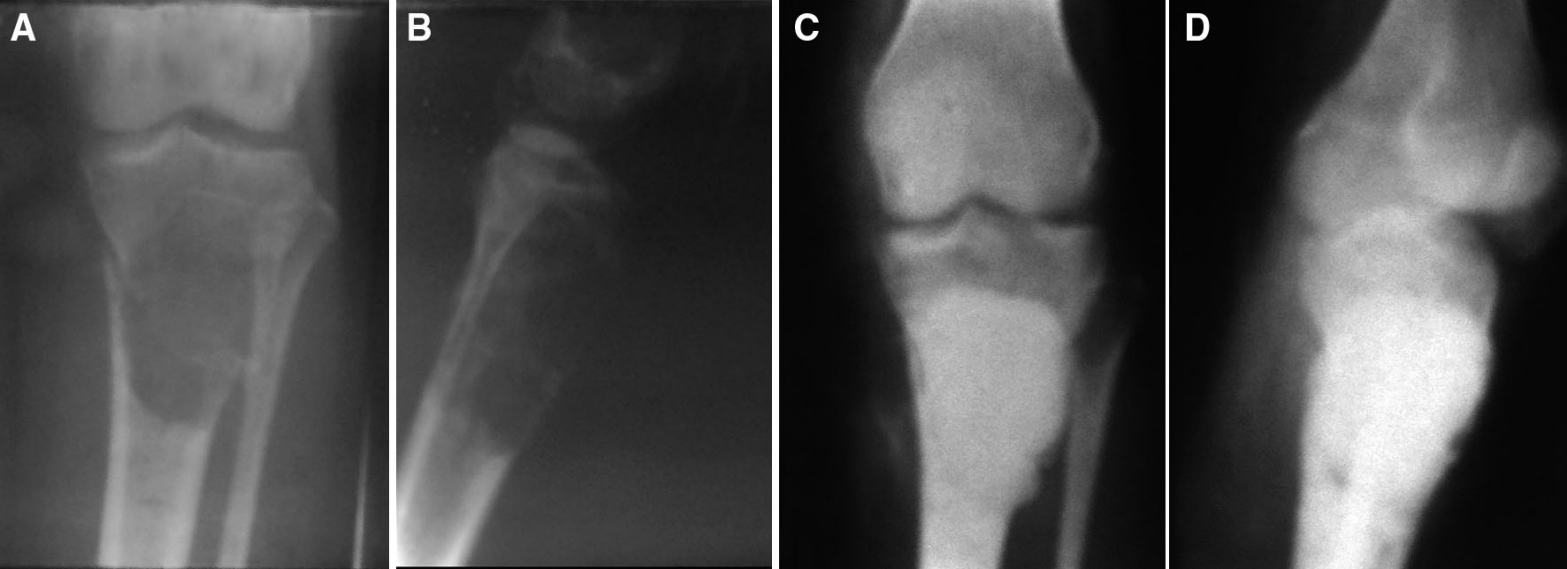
Antero-posterior and lateral radiographs of an 18-year-old male patient showing a giant cell tumor lesion in the proximal tibia with a pathological fracture (a, b). Follow-up radiographs at 14.5 years after curettage and cementation showing good consolidation of the lesion with no recurrence (c, d). The patient had normal range of motion and was asymptomatic
